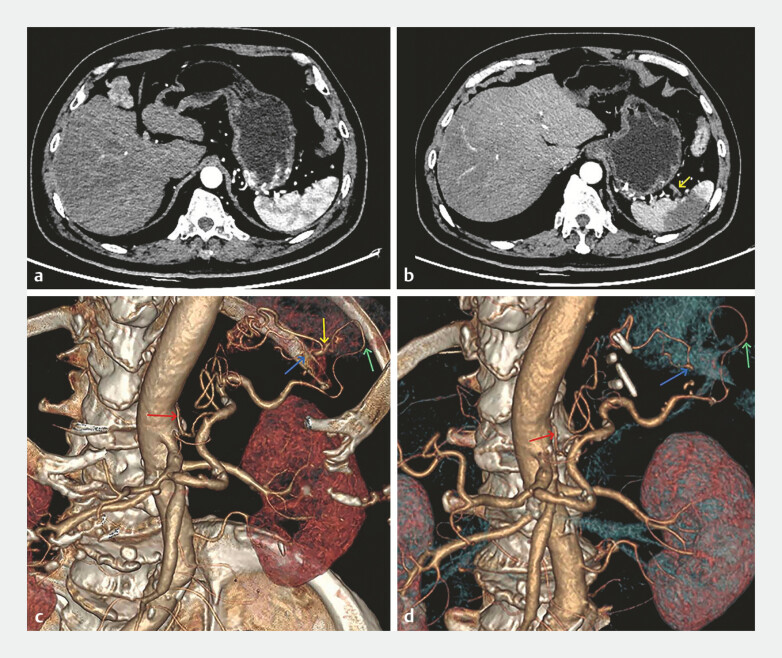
Computed tomography scans (
